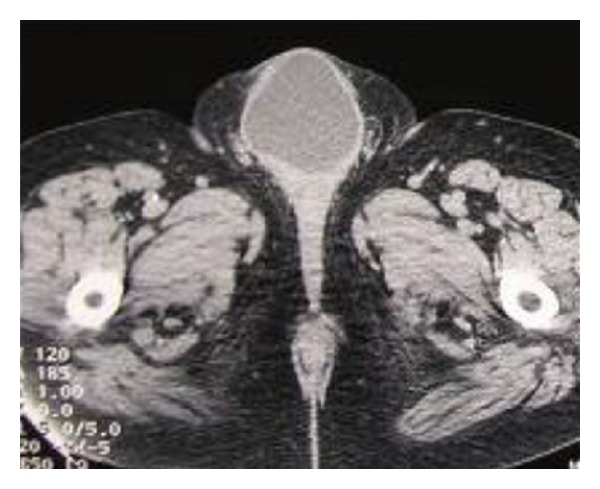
CT of the lower abdomen and pelvis showing a large cystic mass.
